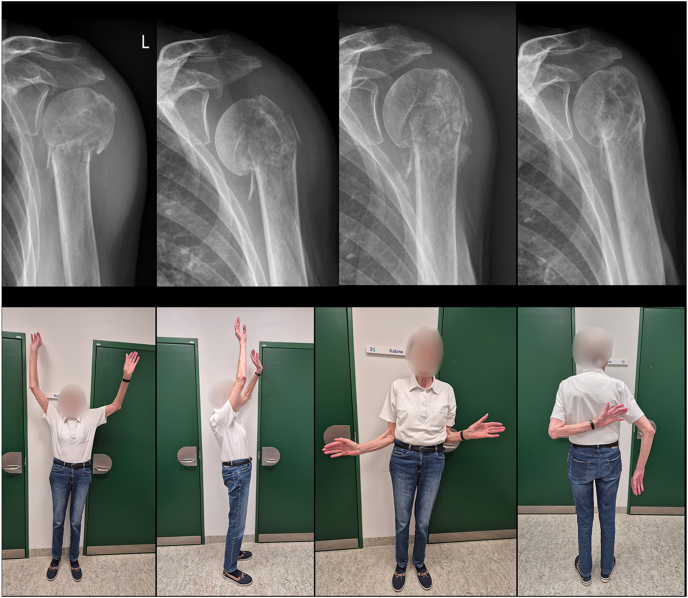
An 82-year-old female with a minimally displaced surgical neck fracture. Varus collapse of the humeral head occurred within the first two weeks post-injury. Partial humeral head resorption was observed during healing. The patient achieved pain-free shoulder function. Anterior–posterior radiographs were taken at admission, 2 weeks, 6 weeks, and 6 months. Photographs were taken after 6 months.
